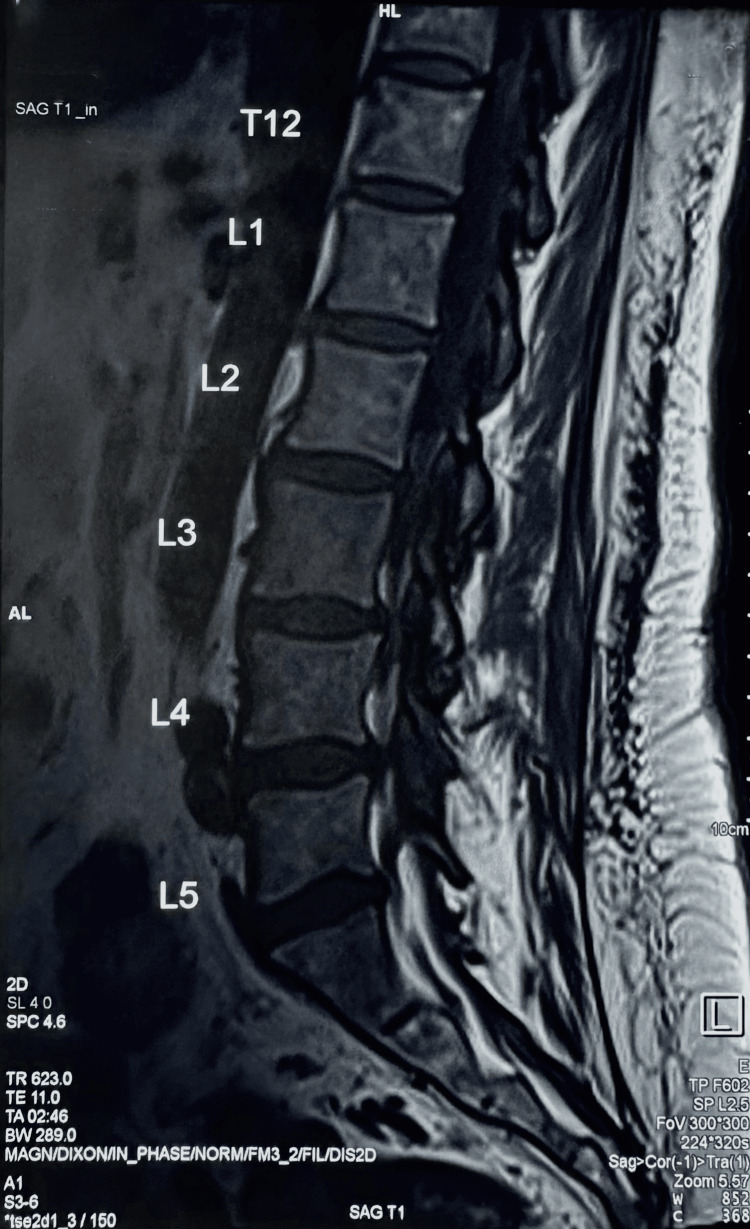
Lumbar MRI Lumbar MRI showing mild spondylotic changes of the lumbar spine. Features are most prominent at L3–4 with minimal narrowing of the spinal canal. No evidence of compression of the cauda equina was observed. MRI: Magnetic resonance imaging
